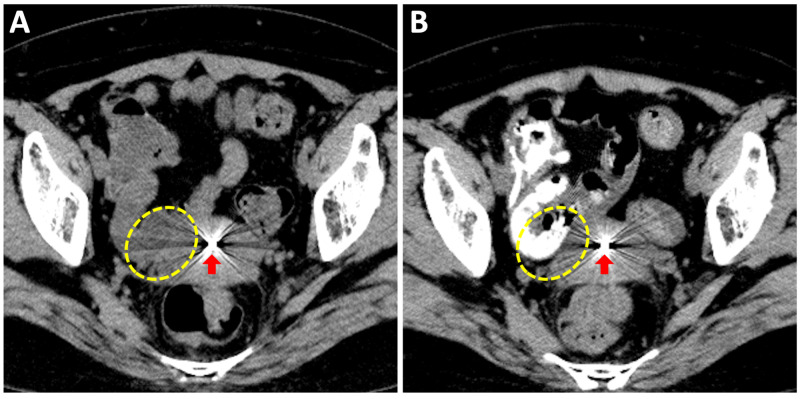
CT images used for treatment planning of brachytherapy obtained in the absence (A) or presence (B) of oral gastrografin pretreatment. The dashed lines show the border regions of the small intestine and the high-risk clinical target volume. The arrow shows the tandem applicator inserted in the endometrial cavity.
